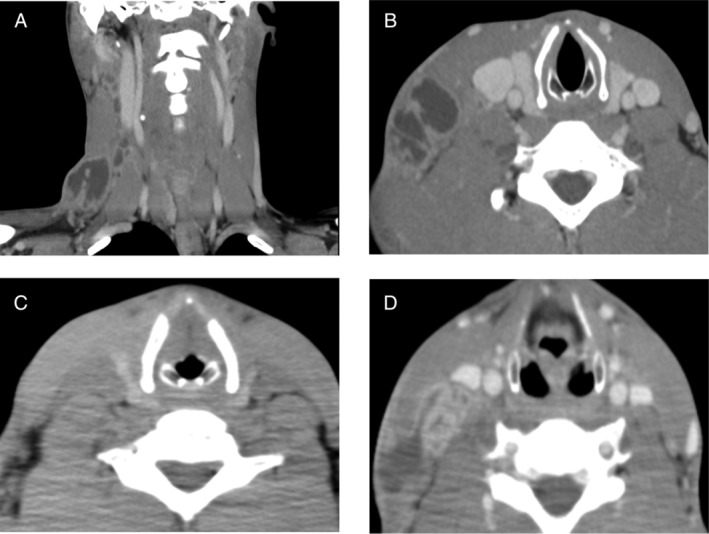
(A, B) Enhanced computed tomography (CT) on the first presentation showed right cervical lymphadenopathy. (C) CT six months after the initiation of anti‐tuberculosis (TB) drugs revealed decrease in the size of the lesions. (D) Enhanced CT obtained one month after treatment completion. Multiple cervical lymphadenopathy was seen in the same place as seen before.
